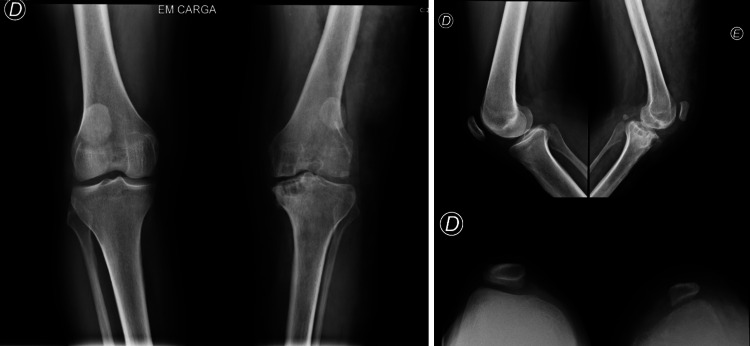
X-rays of the patient’s father’s knees - bilateral patellar hypoplasia associated with osteoarthritis, predominantly on the left side.
